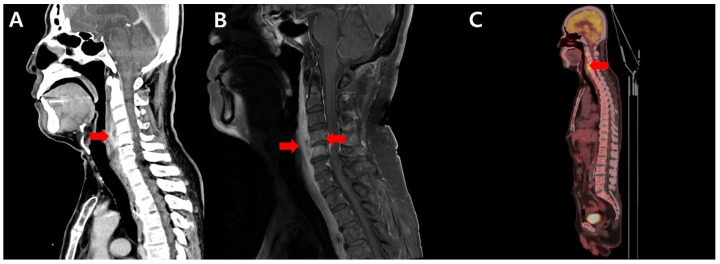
Initial diagnostic imaging of tuberculous spondylitis: (A) Enhanced neck CT demonstrating a hypoattenuated lesion at the C4–5 level with prevertebral soft tissue involvement (red arrow). (B) T1-weighted fat-suppressed sagittal cervical spine MRI showing C4–5 disco-vertebral destruction with prevertebral and epidural infiltrations (red arrows) causing moderate to severe cord compression. (C) PET-CT demonstrating intense FDG uptake around the C4–C5 vertebral bodies and diffuse hyperplastic bone marrow FDG uptake (red arrow).
